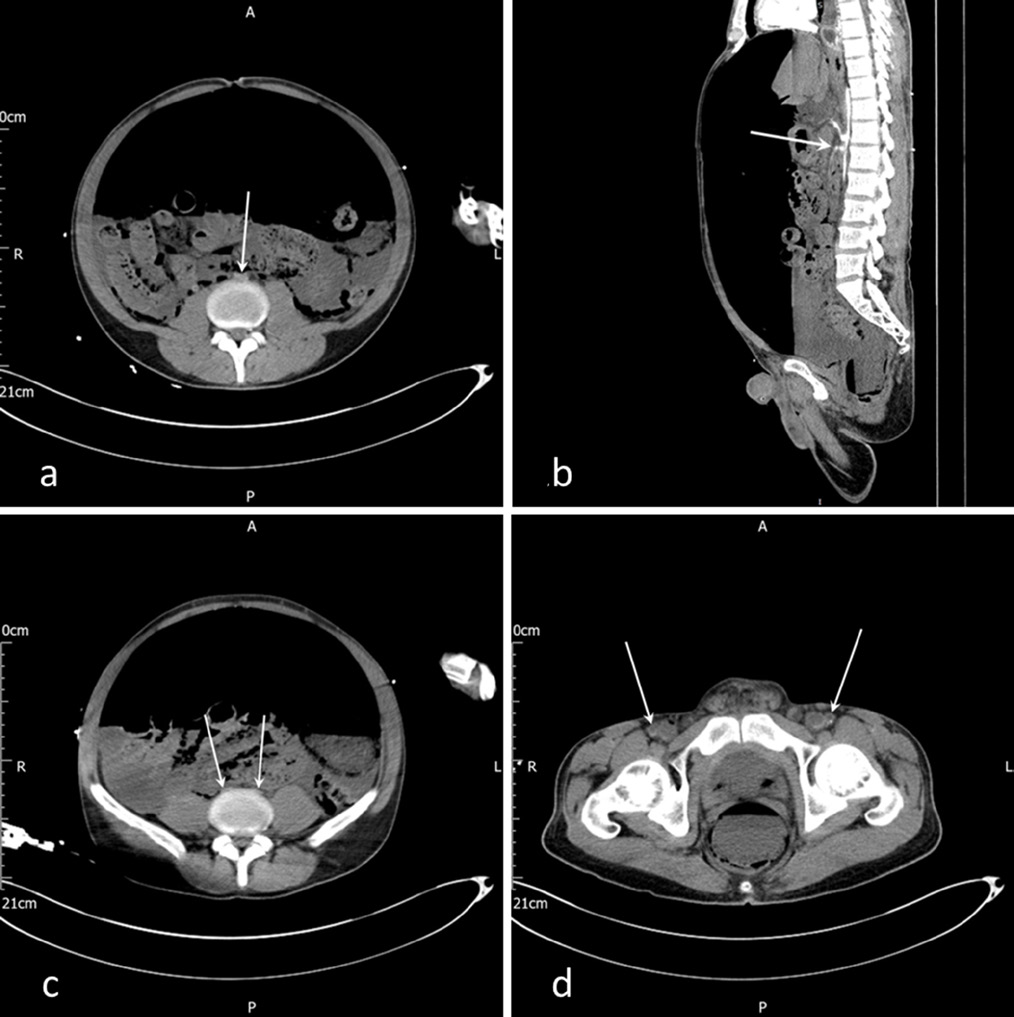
(a) Axial slice of an arterial phase CT scan at the level of the L4 superior endplate with a white arrow showing occlusion of the abdominal aorta (b). Sagittal slice of an arterial phase CT scan demonstrating minimal opacification of the superior mesenteric artery (white arrow) (c). Axial slice of an arterial phase CT, with white arrows demonstrating bilaterally collapsed common iliac arteries (d). Axial slice of an arterial phase CT, with white arrows showing common femoral arteries of normal calibre but with almost no contrast contained within them
